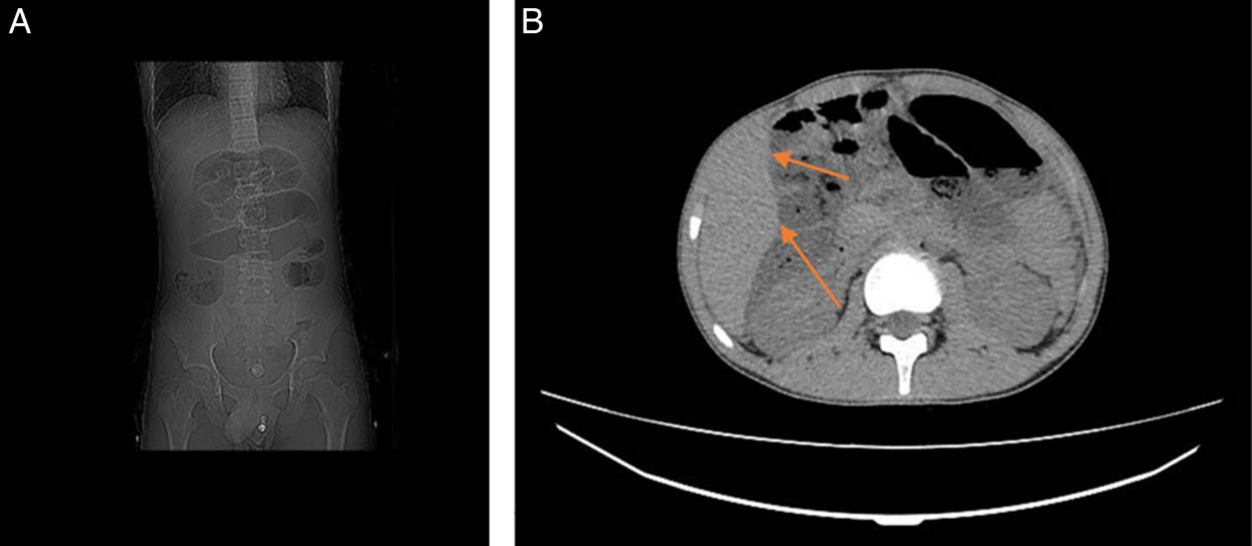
(A) Abdominal radiograph showing multiple fluid-gas levels, (B) Abdominal computed-tomography showing segmental-wall thickening (arrows) with fluid-gas levels.
